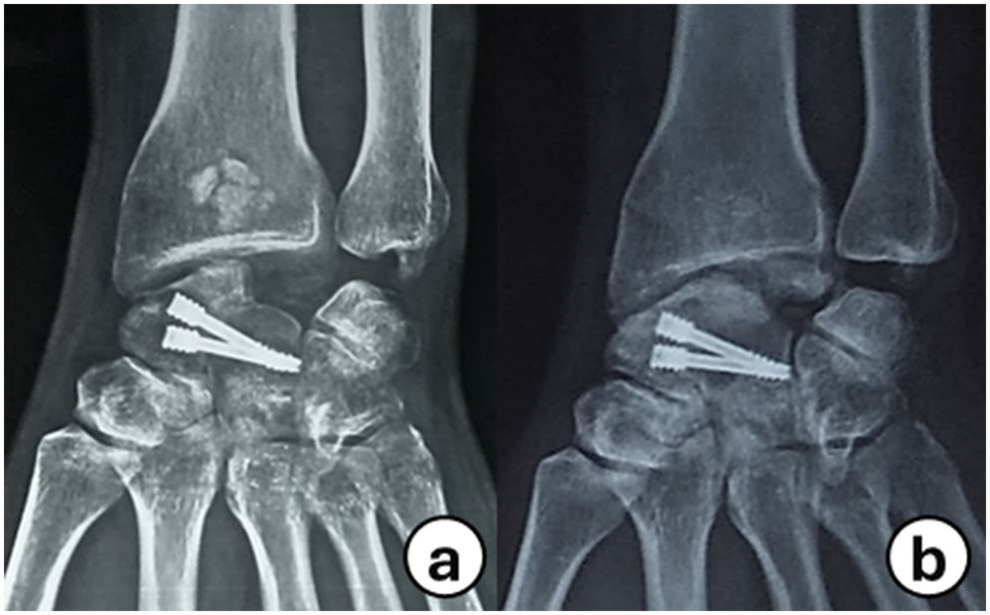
a: Postoperative AP wrist radiograph (4 months) for a 25-year-old male underwent SCF with Lunarectomy, and the excised lunate was implanted at the distal radius harvesting site. b: Final radiograph shows healing of the SCF site along with uptake of the lunate into the distal radius with full consolidation. Evidence of calcification at the site of excised lunate is noted
